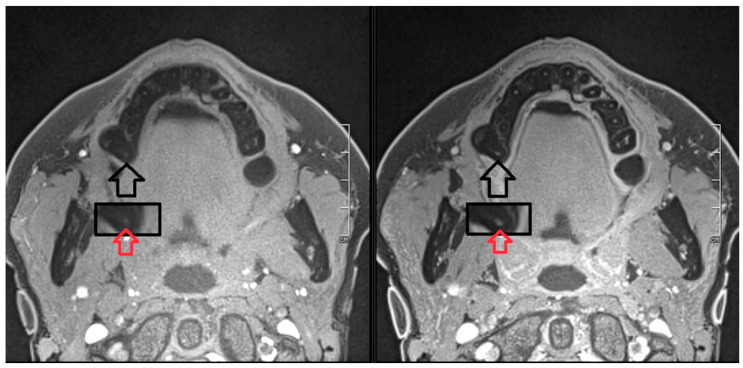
T1-weighted 3T MRI left, without, right, with contrast enhancement in the same patient at our hospital. The enhancement is clearly visible as a white spot in the pulp area after magnification on the right image. The black arrow shows the region of interest, the red arrow on the magnified image shows the pulp without and with contrast enhancement.
